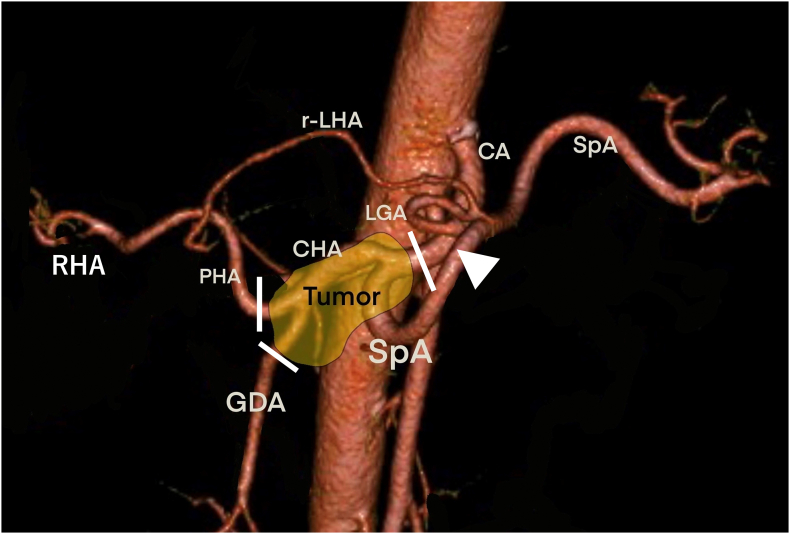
3D-CT angiography. The 3D-CT angiography reveals the existence of r-LHA. The root of the LGA (arrowhead) was not invaded by the tumor. The white lines show transected points of arteries.
